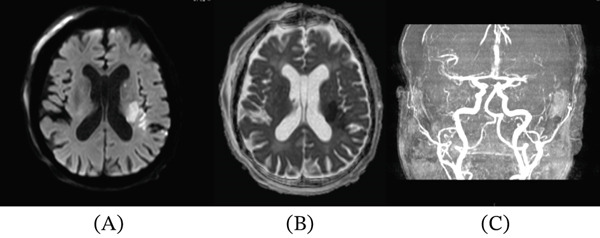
Diffusion‐weighted imaging (DWI) reveals areas of diffusion restriction in the vascular territory of the left middle cerebral artery (A) with corresponding low apparent diffusion coefficient (ADC) values (B), suggesting the acute ischemia caused by the occlusion of the left medial cerebral artery, distal M1 segment/proximal M2 branches with corresponding lack of flow voids on arterial time of flight (TOF) sequence (C).
